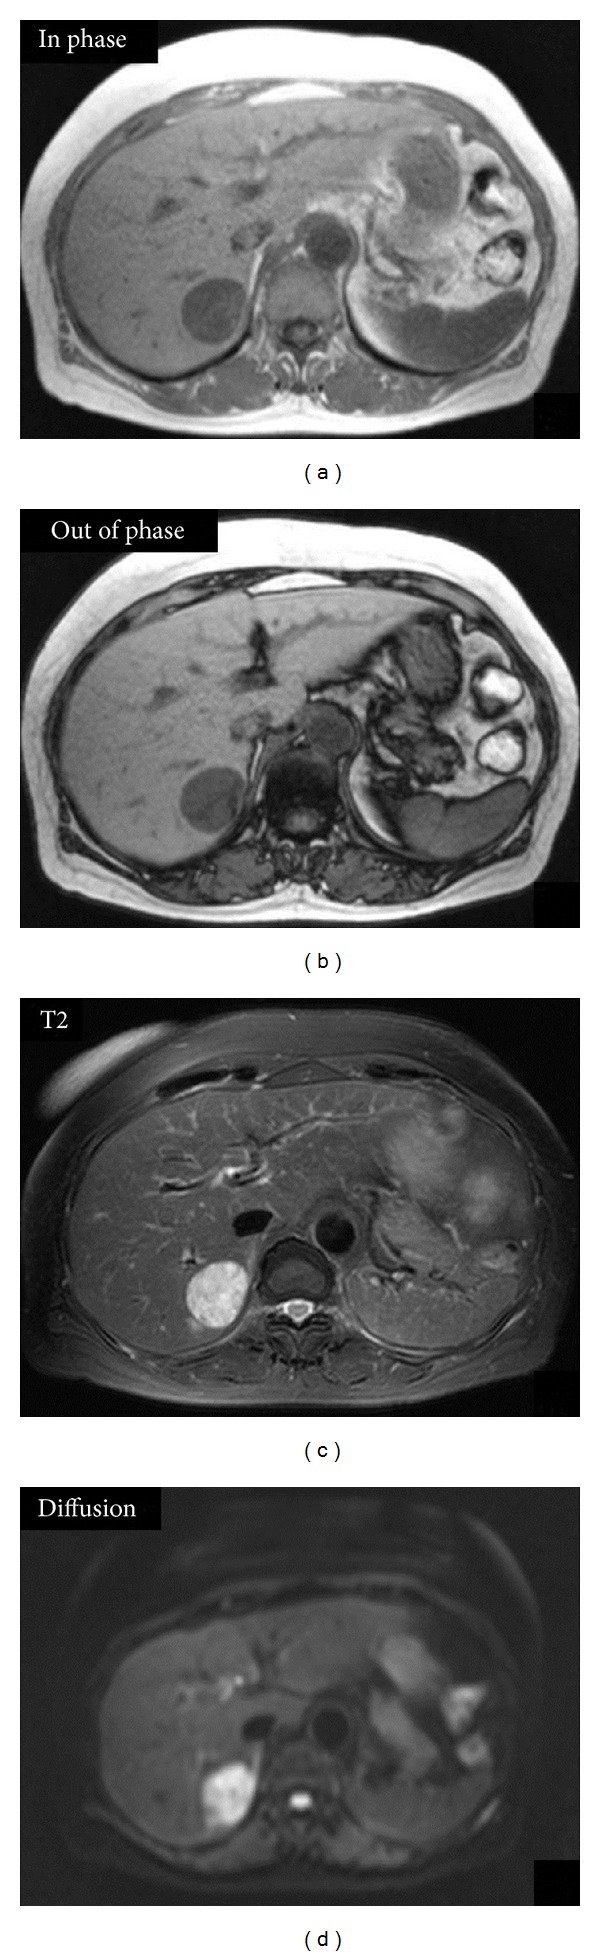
Magnetic resonance imaging. The tumor appeared as a low-intensity area on T1-weighted in-phase (a) and out-of-phase images (b). On T2-weighted with fat saturation and diffusion-weighted images, the tumor appeared as an area of high intensity ((c), (d)).
